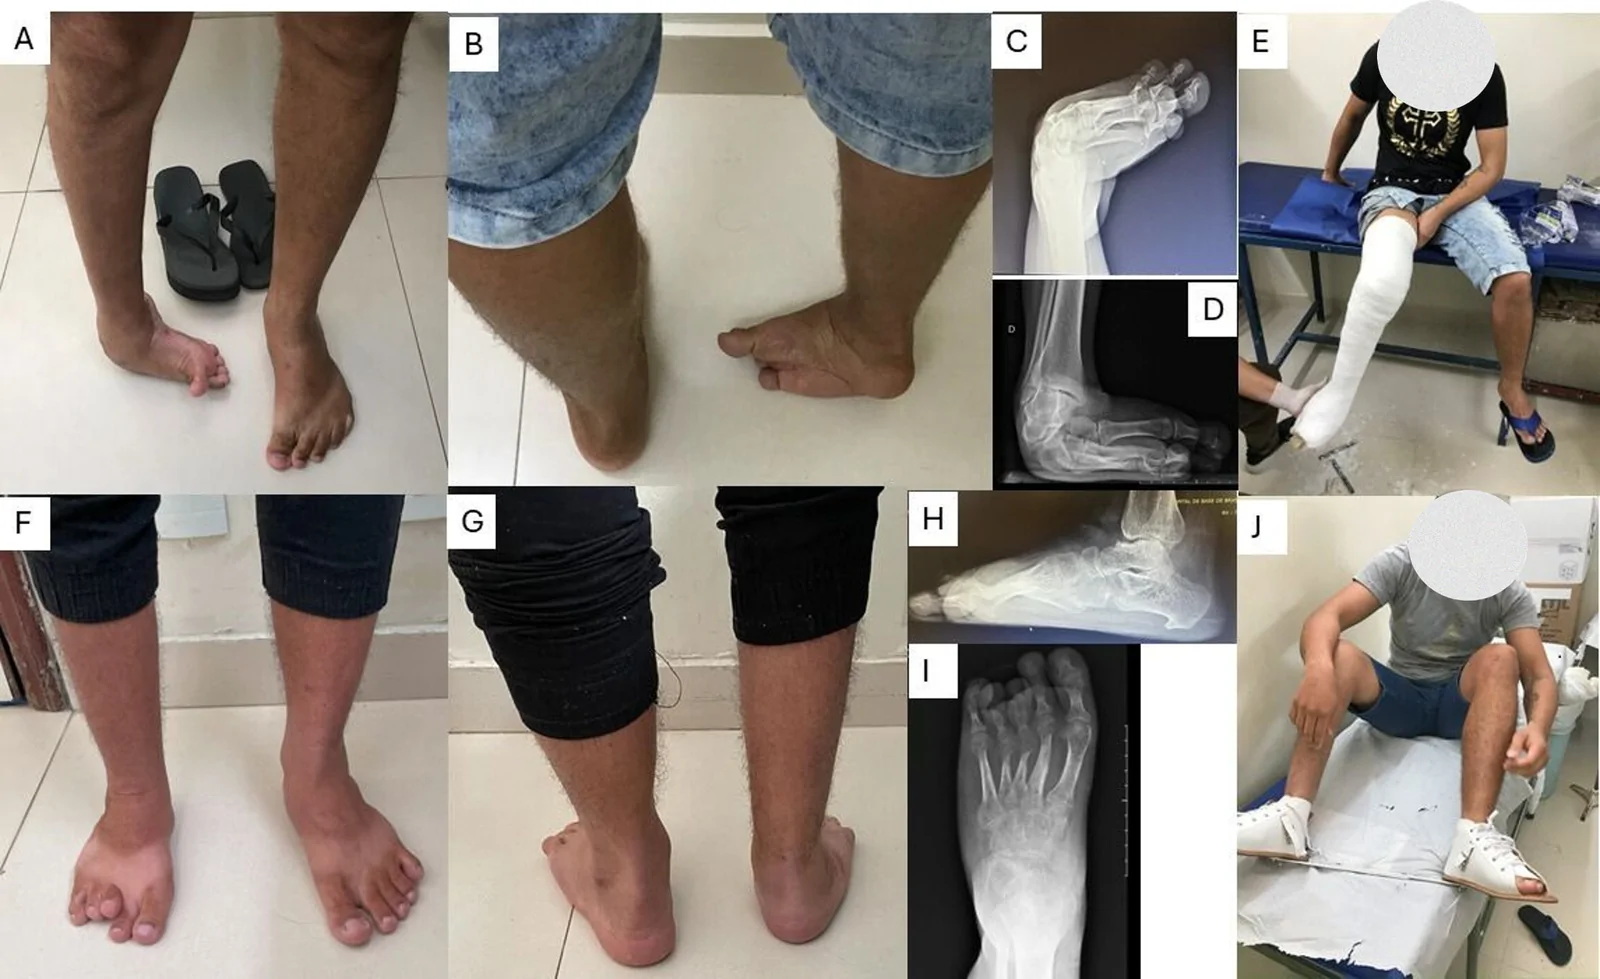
A 22-year-old patient (Patient 16, Table 3). Clinical and radiographic aspects before (A, B, C, D) and after treatment (F, G, H, I). Patient underwent 8 casts (E) and Achilles tenotomy (without any bone surgery). The patient is using the abduction brace after treatment (J).
